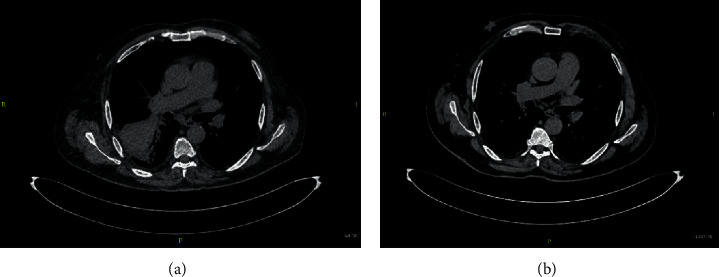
CT image showing pneumonic consolidation in the right lower zone (a) which improved remarkably with treatment 12 months later (b).
